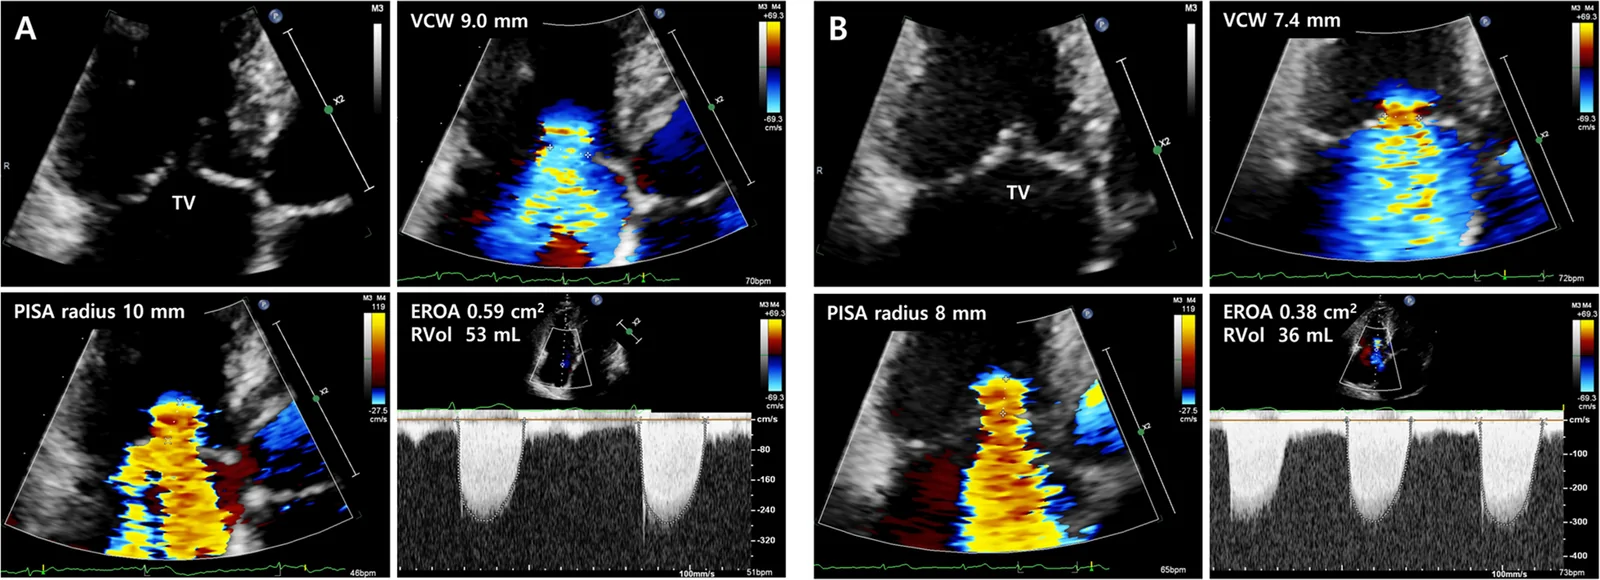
Baseline echocardiography shows tethering of tricuspid valve with severe functional tricuspid regurgitation (TR). Vena contracta width (VCW) was measured, effective regurgitant orifice area (EROA) and regurgitant volume were calculated with proximal isovelocity surface area (PISA) radius, peak velocity and velocity time integral of the TR jet (A). Tethering of tricuspid valve was improved and VCW, EROA, and regurgitant volume were significantly decreased after treatment with empagliflozin for 12 months (B). EROA, effective regurgitant orifice area; PISA, proximal isovelocity surface area; RVol, regurgitant volume; TV, tricuspid valve; VCW, vena contracta width
